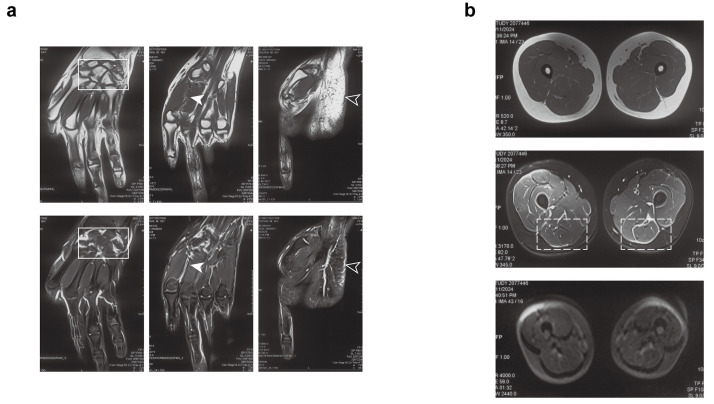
Magnetic resonance imaging (MRI) findings demonstrating musculoskeletal involvement. (a) MRI of the hands and wrists showing ulnar deviation of both wrists, synovial hyperplasia, multifocal bone marrow edema of the carpal bones (boxed areas), peritendinous fluid surrounding the flexor and extensor tendons (solid arrowheads), and soft tissue edema around the wrist joints (open arrowheads). (b) Axial MRI of the lower limbs demonstrating hyperintense signal changes in the fascia and adjacent muscle compartments of the bilateral biceps femoris muscles on fluid-sensitive sequences (dashed boxes), indicating myofascial inflammation; corresponding images without signal abnormalities on non–fluid-sensitive sequences are shown for comparison.
